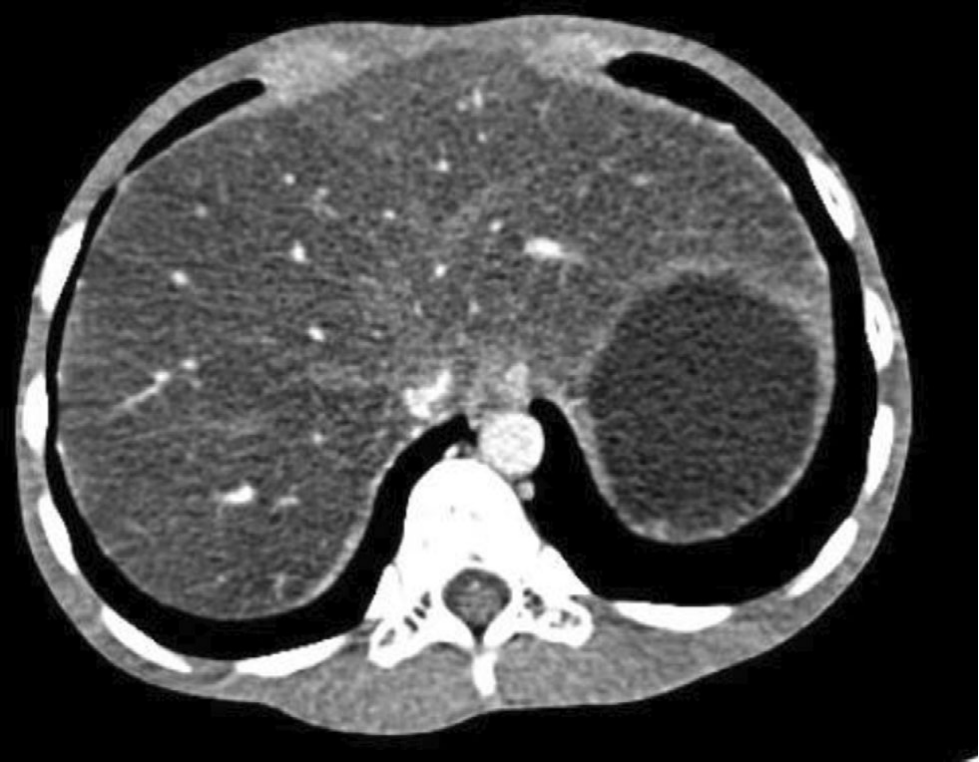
Abdominal CT: increased liver volume and decreased liver parenchymal density and very little subcutaneous fat layer.
